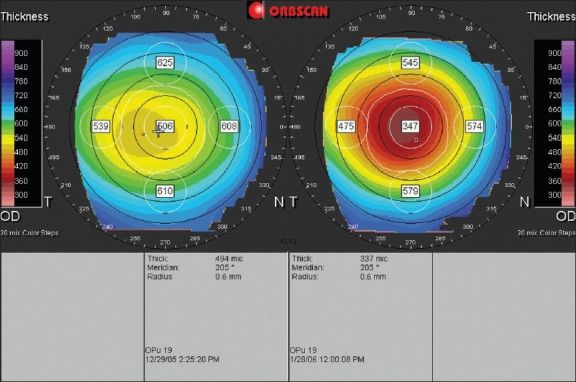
A dual pachymetric display of a right eye before (left) and after (right) keratorefractive surgery for myopia. The preoperative central corneal thickness with the Orbscan was 506 µm, compared to 490 µm with an ultrasound pachymeter (overestimation). After surgery, the values were respectively 347 µm and 395 µm (underestimation)
